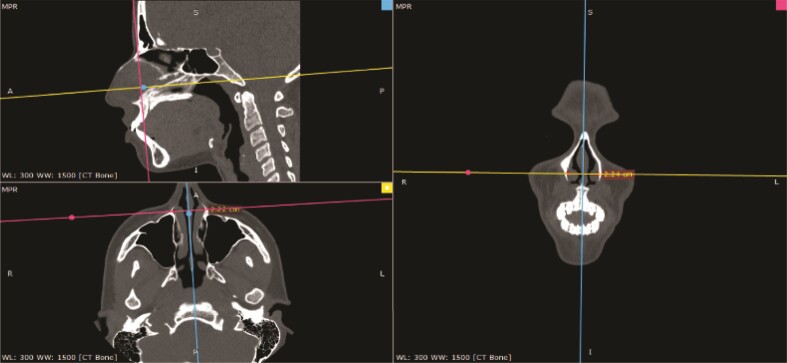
Preoperative evaluation. Preoperative computed tomography scan measure of the distance between inferior turbinate’s heads in Radiant’s software multiplanar reconstruction mode.
